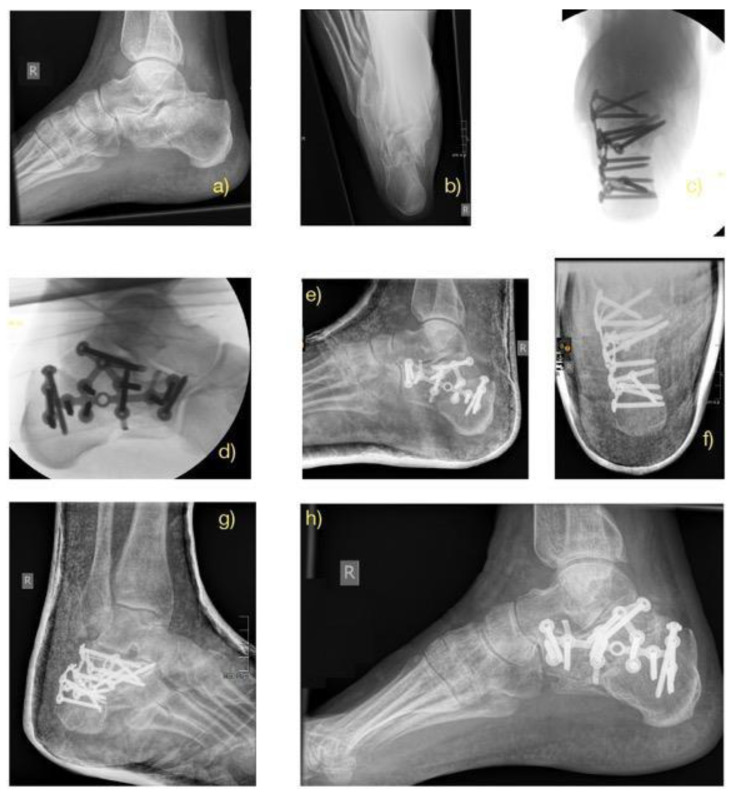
ORIF Case: 62-year-old male patient after a fall on the right calcaneus. Preoperative X-ray lateral (a) and axial (b): intra-articular calcaneal fracture with pathological flattening of the Böhler’s angle and valgization of the right hindfoot. Intraoperative pictures (c,d). Follow-up after 14 days. (e–g) and Follow-up after 12 months (h).
